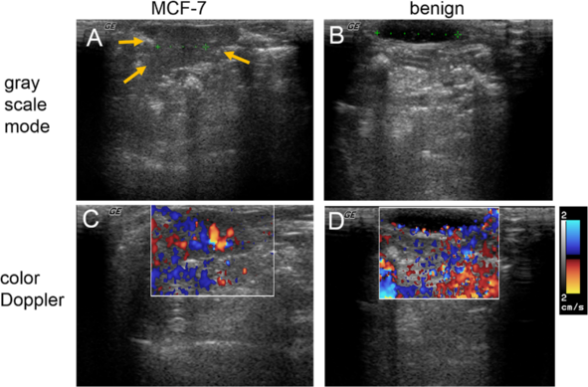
Sonograms and Doppler imaging of developed xenografts in vivo. The longitudinal cross-section images (a, c) were acquired from malignant xenografts. The transverse cross-section images (b, d) were acquired from the benign xenografts. The color Doppler imaging (c, d) quantifies the vascular flow velocity around the xenograft and surrounding region by pseudo colors. The green cursers indicate corresponding xenograft lengths, 0.76 cm in (a) and 1.18 cm in (b). The morphology of malignant xenograft (MCF-7 xenograft) was demonstrated in (a) with angular/ill-defined margin (orange arrows)
